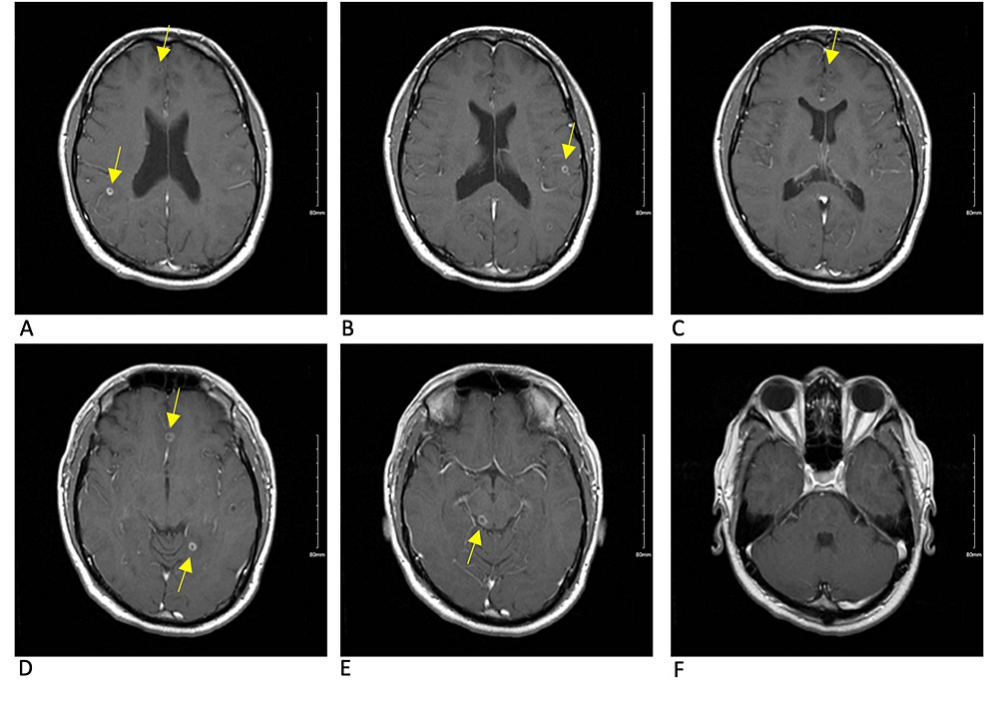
MRI brain T1 axial post-contrast sequence 13 weeks after initial symptom onset This scan depicts the pre-treatment cyst burden as it was performed one day prior to antiparasitic treatment. Overall cyst burden had increased from prior scans with some lesions showing increased edema and some with less. The lesions depicted are continuing to degenerate but remain in the colloidal or granular-nodular stages. The lesion in the anterior right frontal lobe (pane A) has decreased perilesional edema when compared to the prior scan. The anterior left frontal lobe lesion (pane B) seen on the prior scan is absent on this scan. New lesions are seen in the right and left parietal lobes (panes A and B). A significant amount of perilesional edema is seen surrounding a new lesion between the right and left frontal lobes (pane C) and is concerning for subarachnoid involvement. A new lesion is also depicted in the left hippocampus (pane D) with considerable perilesional edema. The posterior right midbrain lesion demonstrates a small decrease in perilesional edema (pane E).
